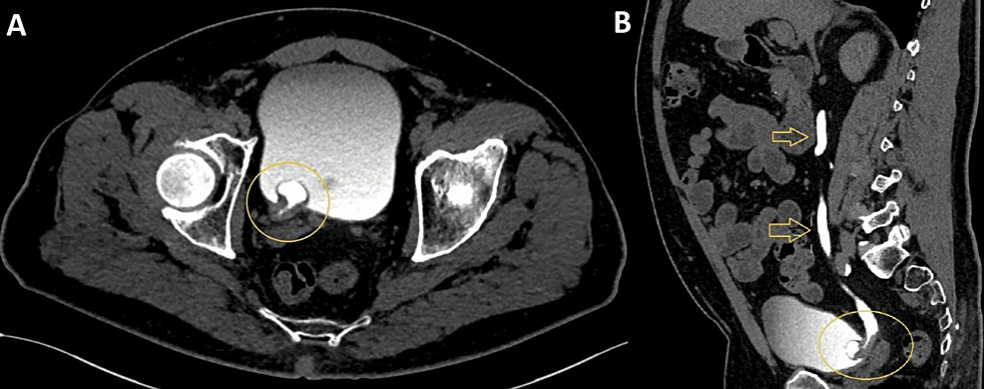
Axial (A) and sagittal (B) contrast-enhanced CT on the excretory phase with a right parietal thickening of a ureterocele (yellow circle) with hydroureter associated (yellow arrows)
